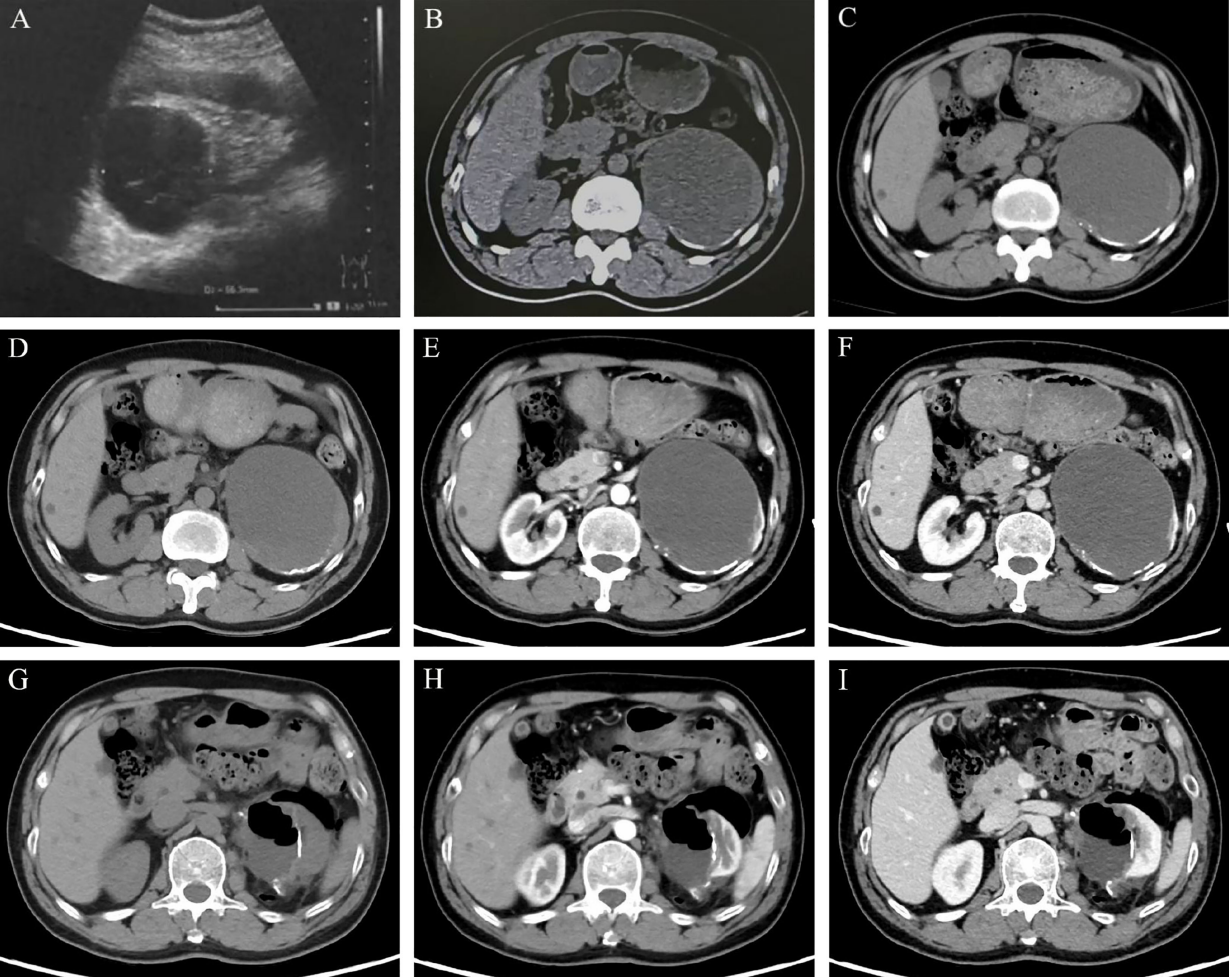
Imaging features of the left renal lesion. (A. February 28, 2013) Ultrasound revealed the cystic echogenic mass measuring 6.4 × 5.5 cm. (B. April 1, 2017) CT scan showed the cystic lesion of 9.5 × 7.6 × 6.7 cm. (C. October 22, 2019) CT scan showed the cystic lesion of 10.0 × 8.2 cm in cross-section with partial cyst wall calcification. Dynamic contrast-enhanced CT was performed to show the features of the lesion in UP (D, G), CMP (E, H), and NP (F, I). (D–F on May 28, 2020) A mixed-density mass dominated by low density was found in the left kidney, with a cross-section of 10.3 × 8.5 cm and multiple high-density patches at the edge. (G–I on June 15, 2020) With localized encapsulated gas and effusion, the left kidney was partially absent accompanied by marginal densification. Abbreviations: UP, unenhanced phase; CMP, corticomedullary phase; NP, nephrographic phase.
